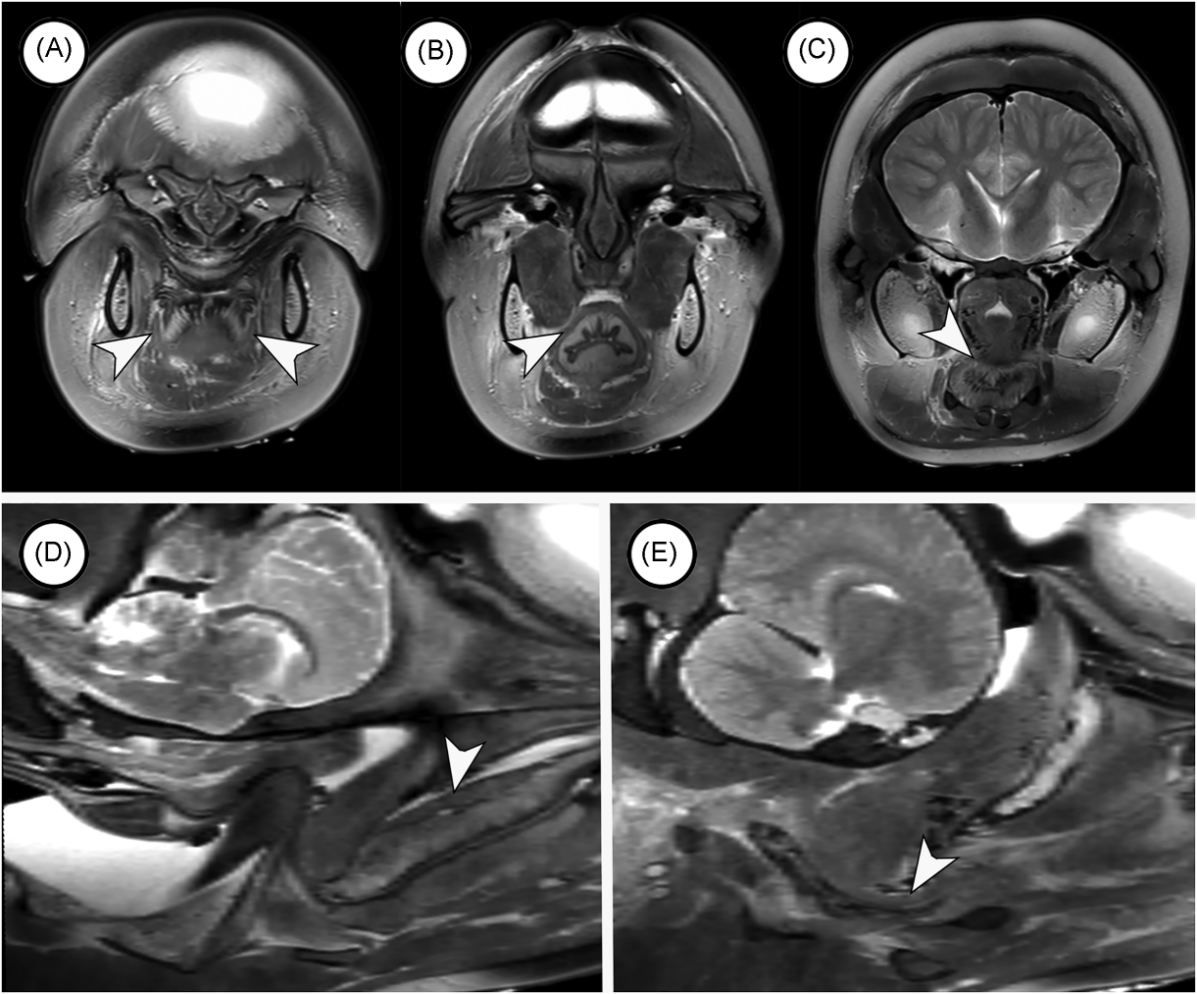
MRI scans in the transverse (A, B, C) and sagittal (C, D) planes showing the distribution of palatoglossus muscles. (A) This scan through the oral cavity shows palatoglossus muscles (arrowheads) on the lateral aspects of the tongue. (B) The palatoglossus muscles become or contribute to a muscular layer that surrounds the oropharynx. (C) This muscle layer merges with the sphincter in caudal regions of the oropharynx. (D) In this midsagittal scan, the muscle layer (arrowhead) associated with the dorsal aspect of the oropharynx merges caudally with the sphincter. (D) In a more lateral sagittal scan, the muscle layer (arrowhead) around the oropharynx merges with the sphincter.
